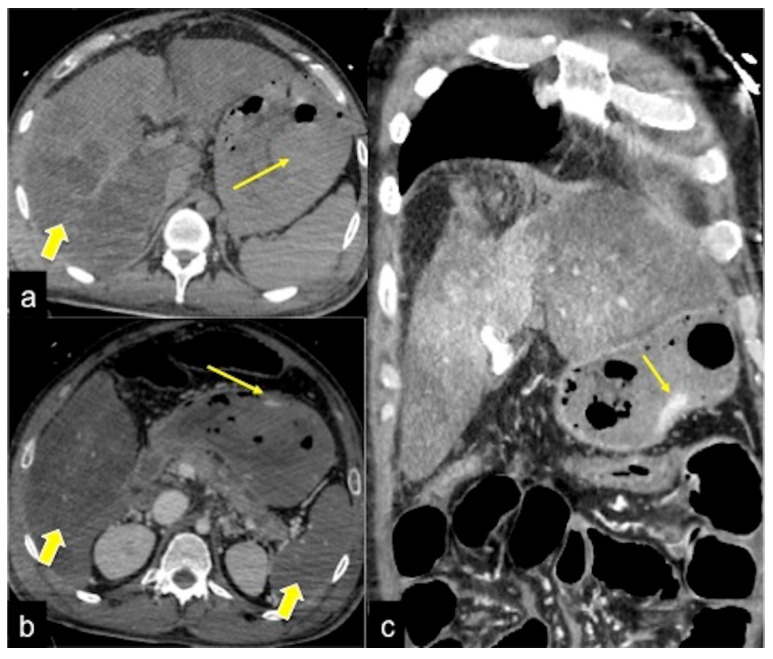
CTA multiphasic study of gastric bleeding in hypopefusive complex. The pre-contrast phase (a) shows a gastric endoluminal area of spontaneous hyperdensity (long arrow) as a sentinel clot associated with alteration of liver density in the right lobe (large arrow). In the arterial phase (b) an active haemorrhagic focus in the stomach (long arrow) associated with hypo perfusion of the liver and splenic parenchyma (large arrows) are detected as signs of hypotensive general status. In the venous phase (c) there is an enlargement of the haemorrhagic blush (long arrow) with inhomogeneous liver perfusion.
